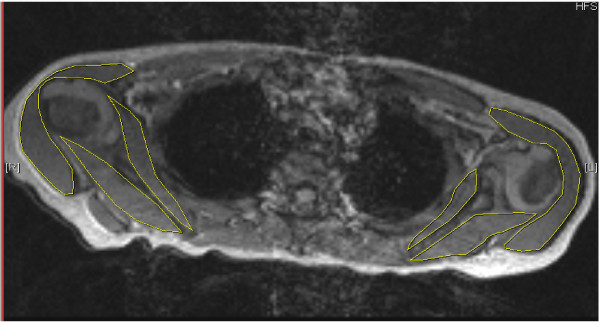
FISP acquisition MRI in axial plane showing affected and normal contralateral shoulder. In the affected left [L] shoulder there is a biconcave glenoid form (type 3) and humeral head subluxation. The contralateral [R] shoulder is normal. Measured areas of infraspinatus, subscapularis and deltoid are outlined.
